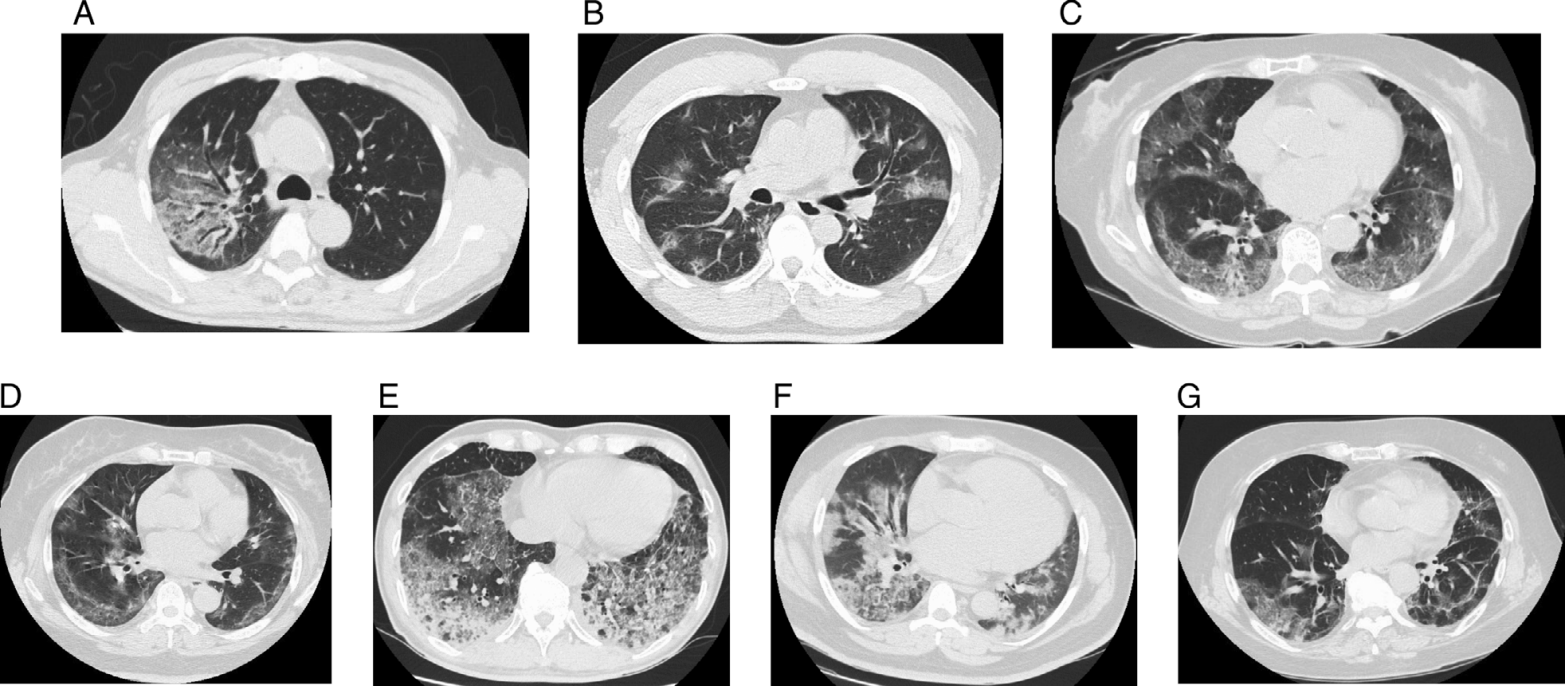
Unenhanced computed tomography images of seven patients on admission day. A–G corresponds to Case 1–7, respectively.
